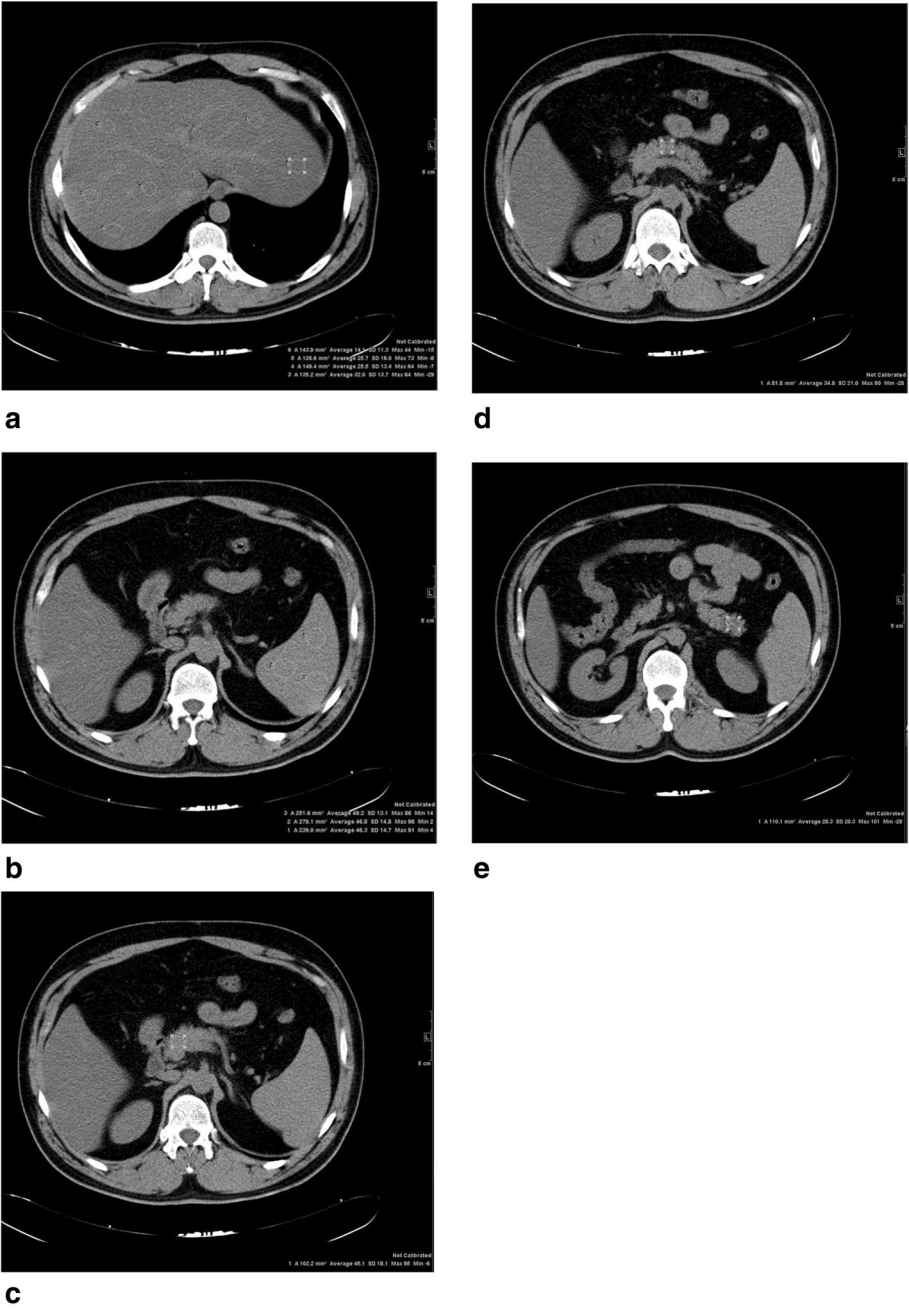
Images of a 35-year-old male who is a candidate for liver donor with hepatostaetosis. (a–e) Unhanced transverse CT images are shown. HU was measured with ROI from liver, pancreatic head, body, tail and spleen. Mean pancreatic and splenic CT attenuation was 37 and 47 HU, respectively. Difference between pancreatic and splenic attenuation (P-S) and pancreas-to-spleen attenuation ratio (P/S) were − 10 HU and 0.79. Pancreatic steatosis grade 2 was found according to the parameters used. (P_mean_, P-S, P/S). The liver attenuation index was found <  − 10 and was accepted as steatosis > 30%.
